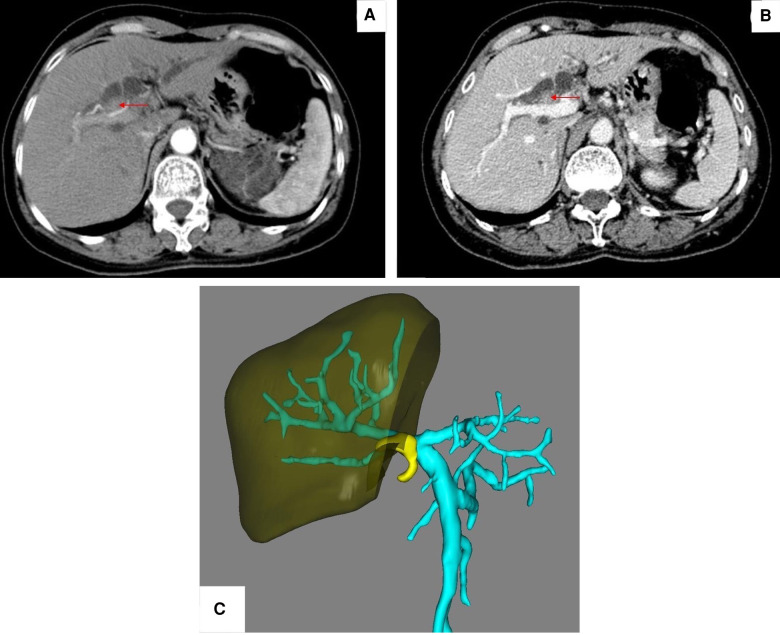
(A) CT revealed a 10 × 17 mm space-occupying lesion (red arrow) in the hepatic hilum with an unclear boundary. (B) Tumor (red arrow) showed obvious enhancement. (C) Postoperative left residual volume was estimated to be only 27.6%.
